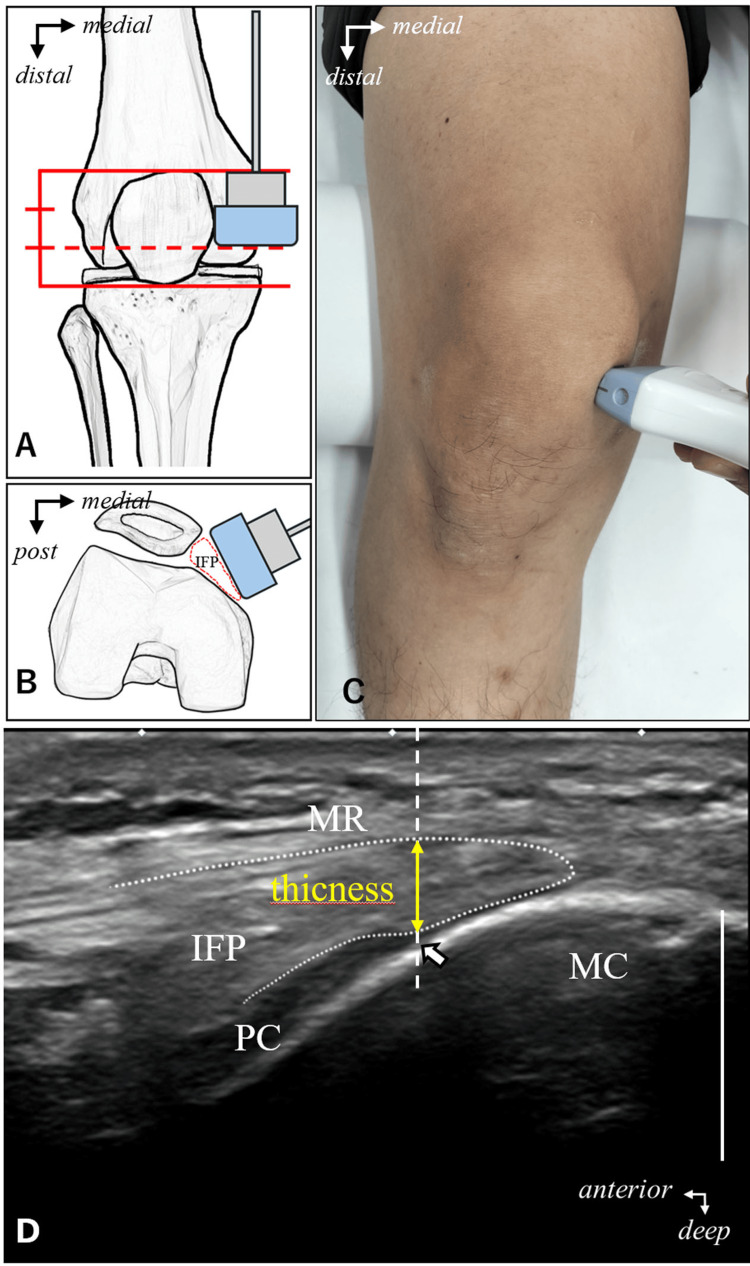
Imaging method for medial IFP thickness. A: a line was drawn medially at the distal 1/3 of the length of the long axis of the patella to determine the imaging position. B: a short-axis ultrasound image was taken with the probe placed between the patella and the tibia. C:  shows the actual imaging. The same method was used for the outer IFP thickness, and imaging was performed at the lateral part of the patella. D: shows medial IFP thickness measurement method. A vertical line was drawn at the border between the medial femoral condyle and the articular cartilage of the patellar surface, and the thickness of the IFP was measured at that point. Arrows indicate the medial edge of the articular cartilage of the patellar surface. MC: medial condyle; PC: patellar surface cartilage; MR: medial patellar retinaculum; IFP: infrapatellar fat pad. Scale bar 10 mm.
